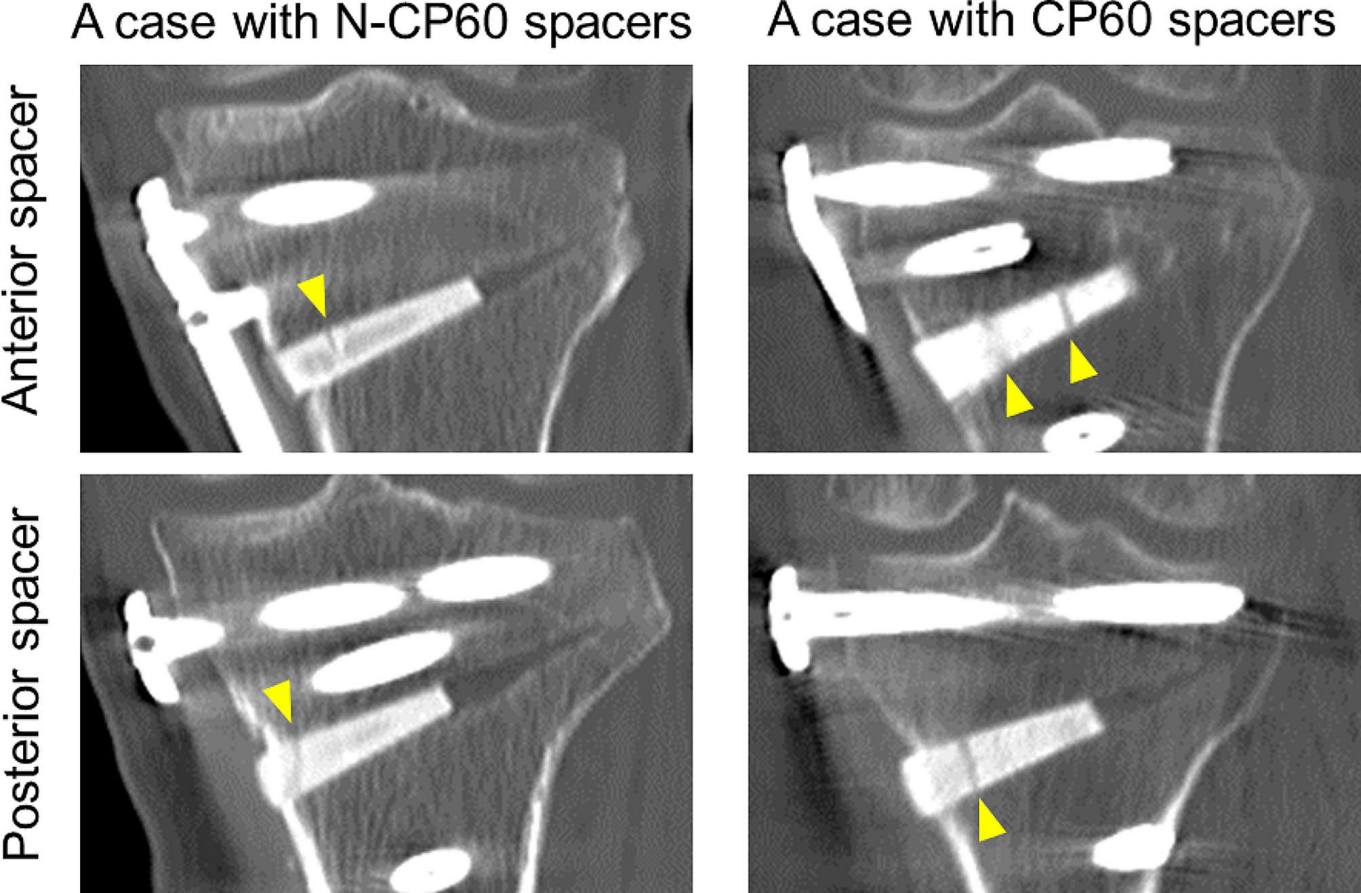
CT images of cracks (indicated with yellow arrows) in a representative case with N-CP60 or CP60 spacers. In each patient, 2 spacers were implanted anteriorly and posteriorly in the opening space of the tibia
